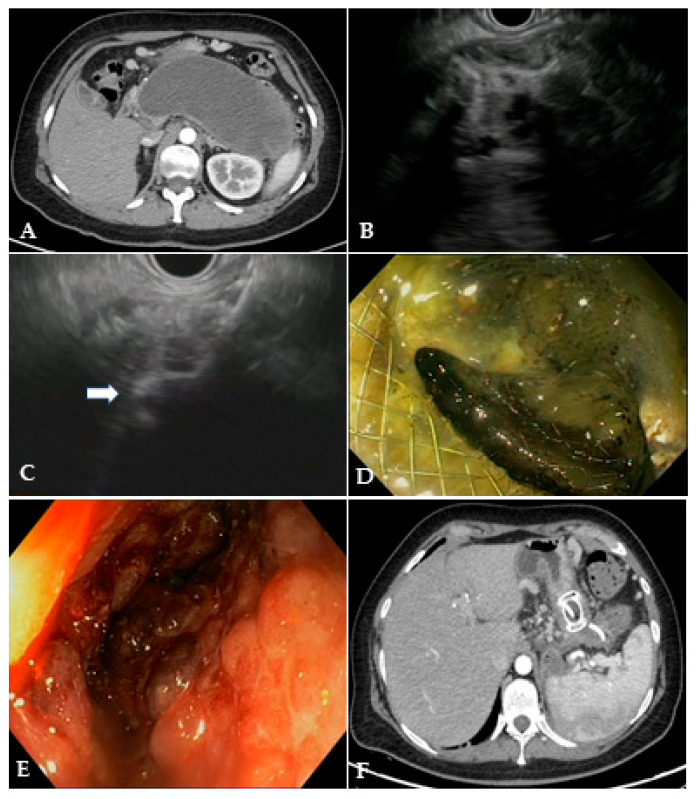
(A) CT scan and (B) EUS image of a large WON with large amount of solid necrotic material; (C) EUS image of the distal flange of a 20 mm LAMS (white arrow) deployed into the WON; (D) endoscopic image through the LAMS of the necrotic cavity completely filled with necrotic debris; (E) endoscopic image of the clean cavity after drainage and repeated necrosectomy sessions; (F) CT scan image of the collapsed cavity with indwelling LAMS and a 10 French/4 cm coaxial DPS. CT: computed tomography; EUS: endoscopic ultrasound; WON: walled-off necrosis; LAMS: lumen-apposing metal stent; DPS: double-pigtail stent.
